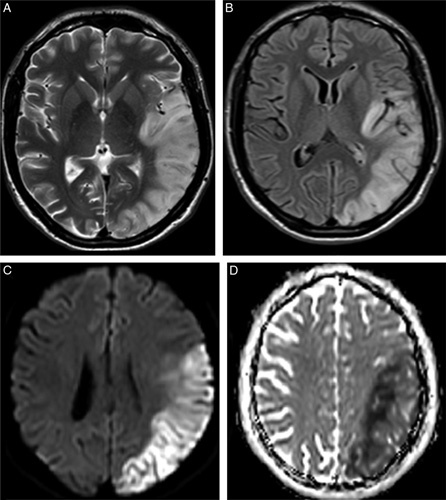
MRI Brain showing T2/FLAIR hyperintensities with extension of infarct in the occipital region with diffusion restriction.
